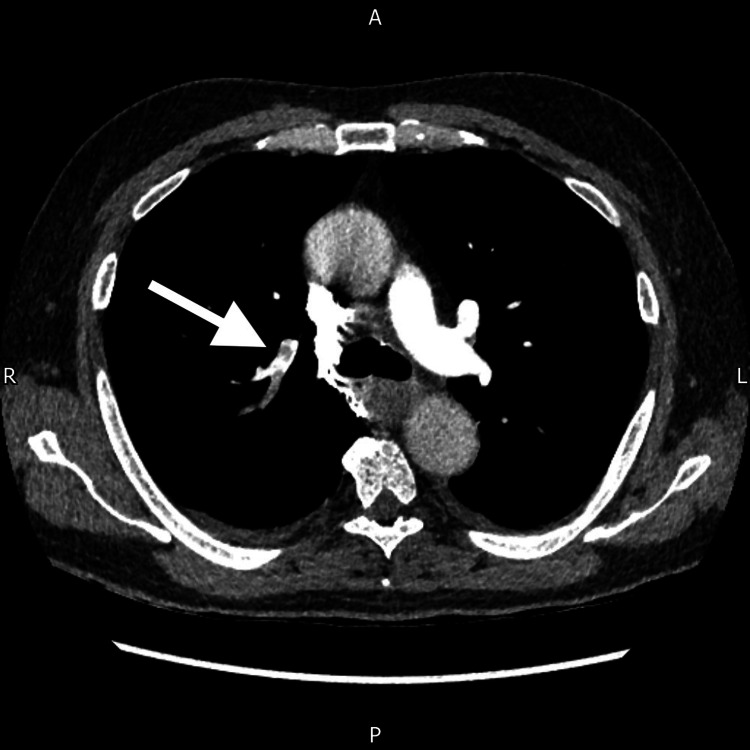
CT pulmonary angiogram, shown in an axial cut, demonstrating a pulmonary embolus in the right lung
